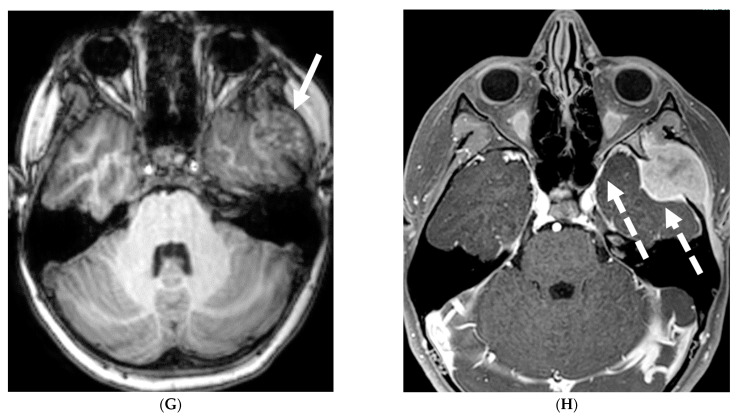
Axial CT bone (A) and brain (B) windows, Axial T2 (C), Axial FLAIR (D), Trace DWI (E), ADC (F), Axial T1 pre (G) and post contrast (H): 9-year-old boy with palpable left cheek mass. There is an ovoid destructive mass (white arrows) in the greater sphenoid wing bulging into the middle cranial fossa with heterogeneous slight restricted diffusivity. The lesion avidly enhances with dural thickening and enhancement (dashed arrows) extending toward Meckel cave and the cavernous sinus. Pathology: LCH.
